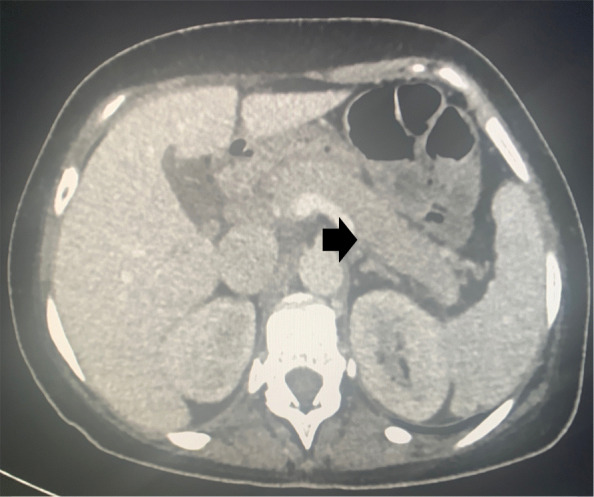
CT Abdomen*.* A CT image of the abdomen*.* The pancreas appears diffusely oedematous (particularly noticeable in the body and tail [black arrow]) with very mild surrounding inflammatory stranding
